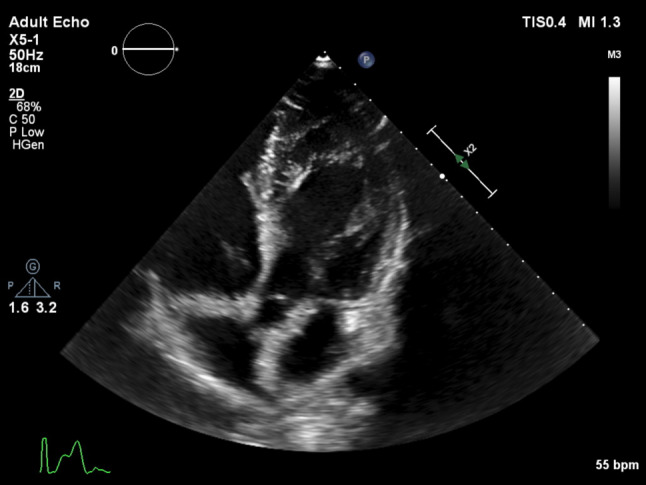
Transthoracic echocardiogram. Apical 5‑chamber view demonstrating prominent left ventricular false tendon from the septal base to the apex
